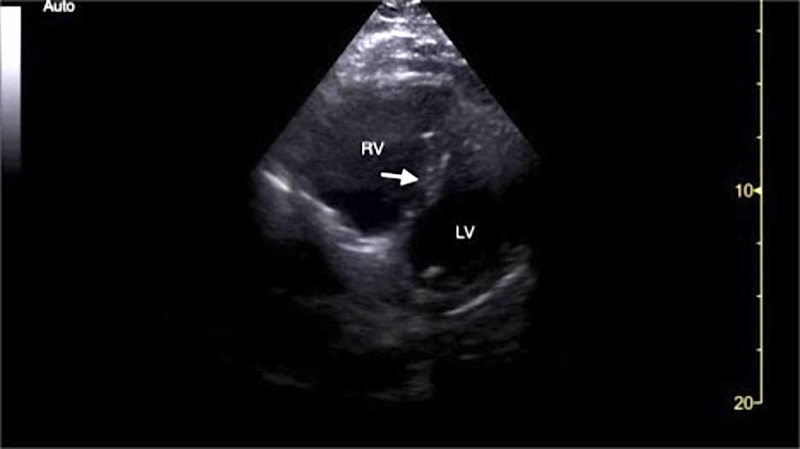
Point-of-care echocardiography pre-thrombolysis. Parasternal short-axis view demonstrating RV dilatation and interventricular septal flattening (white arrow). LV, left ventricle; RV, right ventricle.
